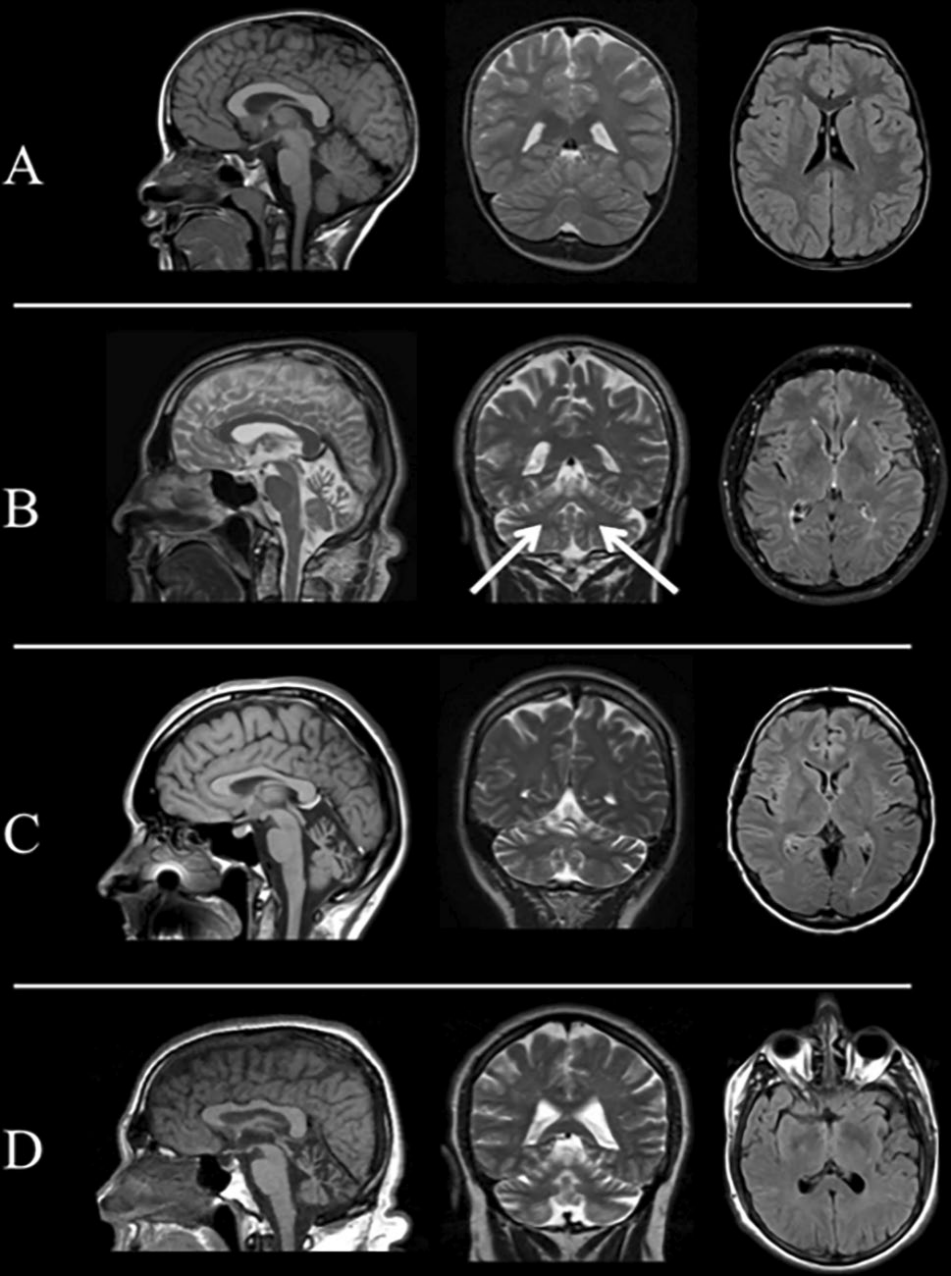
MRI: Representative brain MRI findings in SCA 14 patients. Brain MRI scans of four SCA14 patients (A: Pt. 13; B: Pt. 2; C: Pt. 8; D: Pt. 1). In the first two columns, from left to right, T1-weighted sagittal (with the exception, in B of a T2-weighted sequence) and coronal T2-weighted images showing the absence (A), the presence of moderate (B; C) and severe (D) cerebellar atrophy, respectively. In the third column axial Fluid Attenuated Inversion Recovery (FLAIR) images showing the absence of supratentorial signal changes, as well as preserved cerebral volumes (finding also visible in the other columns). The arrows in B indicate the presence of a mild T2-weighted hyperintensity affecting both dentate nuclei
